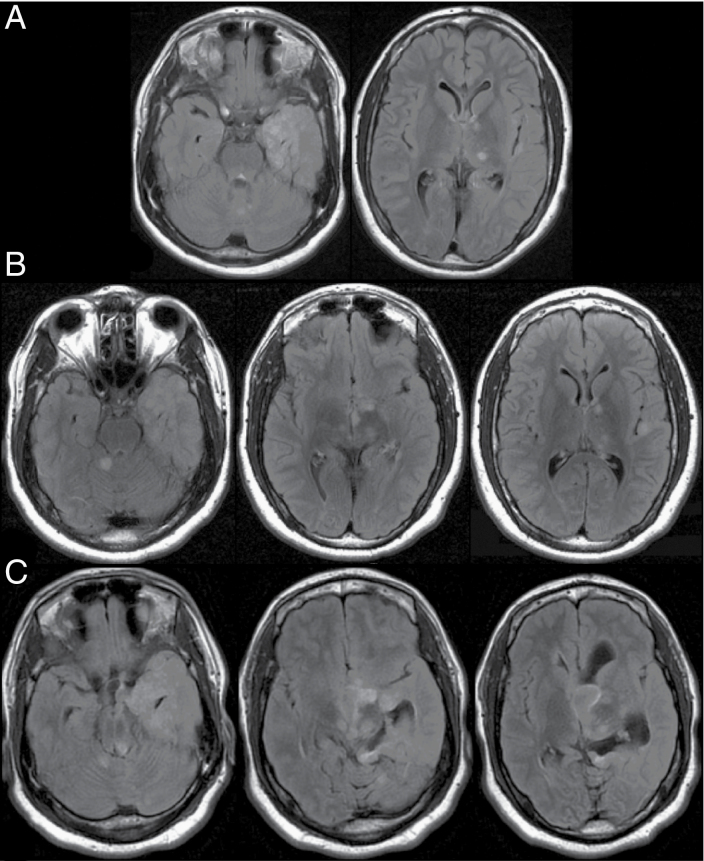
Axial Flair MRI demonstrating RGNT over a 6-year surveillance period. (A) 2013 MRI showing left temporal lobe cortical abnormalities with extension into the deep gray-white matter. (B) Surveillance MRI taken in 2016 that demonstrated stable cortical lesions with slight increase in the T2 signal in the superior cerebellar vermis. (C) MRI in 2019 at presentation to our institution with dramatic expansion of the previously stable left temporal lobe lesion with involvement of the left gangliocapsular region, bilateral thalami, tectum, and cerebellum. In addition, a new non-enhancing cystic component within the left foramen of Monro and third ventricle was identified, resulting in acute obstructive hydrocephalus.
